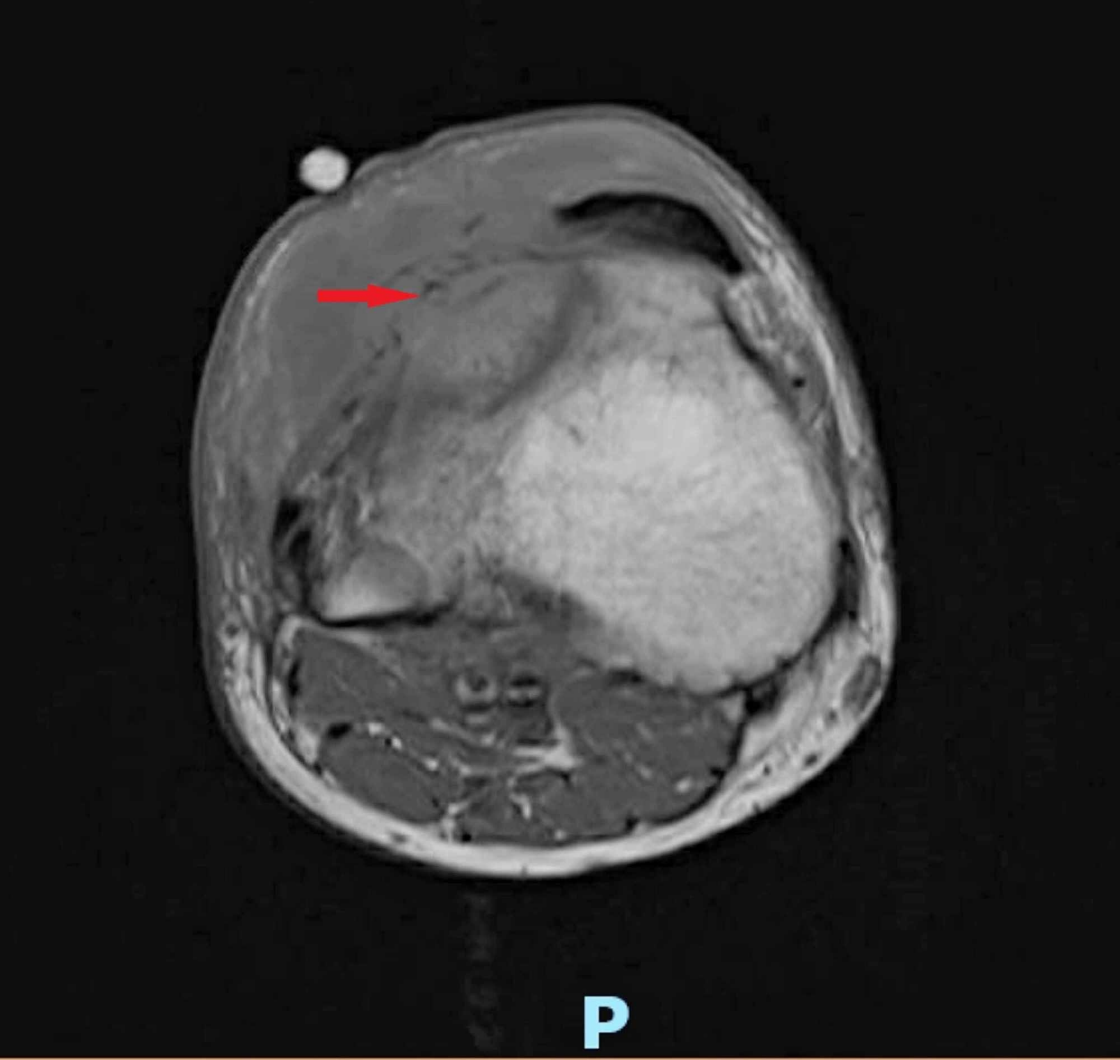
Magnetic resonance imaging (MRI) scan of the right knee shows osteomyelitis involving the lateral tibial plateau, extending inferiorly into the tibial tubercle, along with a 6 x 2 cm abscess within the subcutaneous tissues anterolaterally at the level of the proximal tibia (red arrow)
